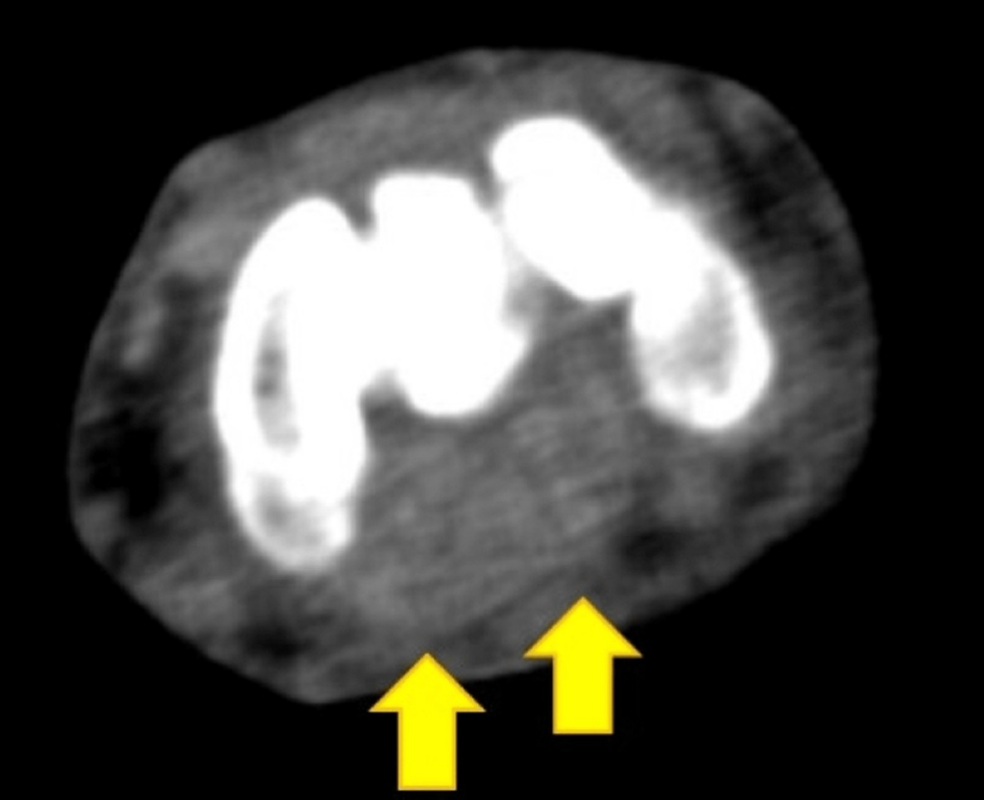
Contrast-enhanced CT of the patient The image showed a low-density area spreading around the flexor tendon, suggesting synovial hyperplasia, from the distal radioulnar joint to the carpal bone level CT: computed tomography
